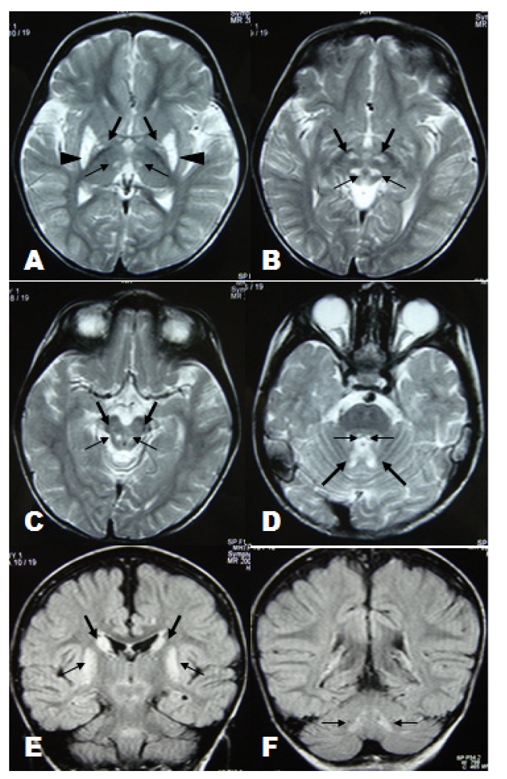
(A): Axial T2-weighted image through the basal ganglia shows symmetrical hyperintense lesions involved thalamic posteromedial ventral nuclei (thin arrow), globus pallidi (thick arrow) and putamina (arrowhead). (B-D): Axial T2-weighted images through the brainstem show symmetrical involvement of reticular formation of midbrain (thin arrow in B), subthalamic nuclei (thick arrow in B), substantia nigra (thick arrow in C), dorsal midbrain (thin arrow in C) central tegmental tracts (thin arrow in D) and cerebellar nuclei region (thick arrow in D). (E-F): Coronal FLAIR images show symmetrical involvement of head of caudate nuclei (thick arrow in E), putamina (thin arrow in E) and dentate nuclei (thin arrow in F).
